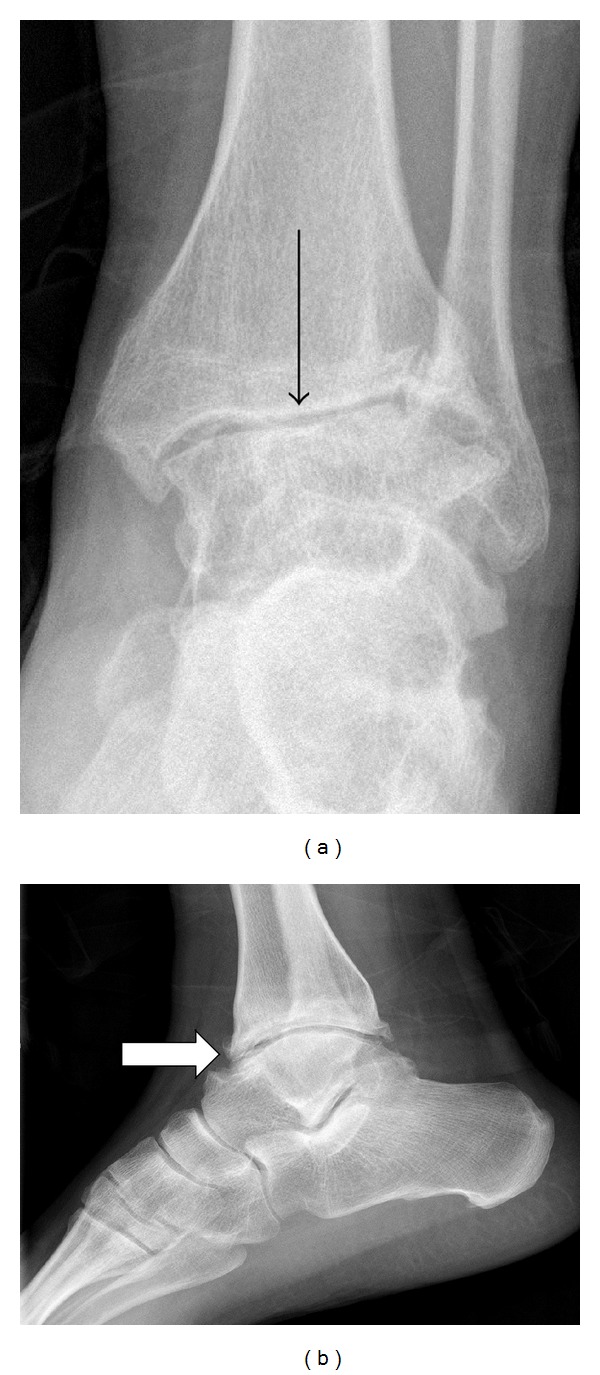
Hemophilic arthropathy of the ankle (black arrow) in the AP (a) and lateral (white arrow) radiographs (b).
